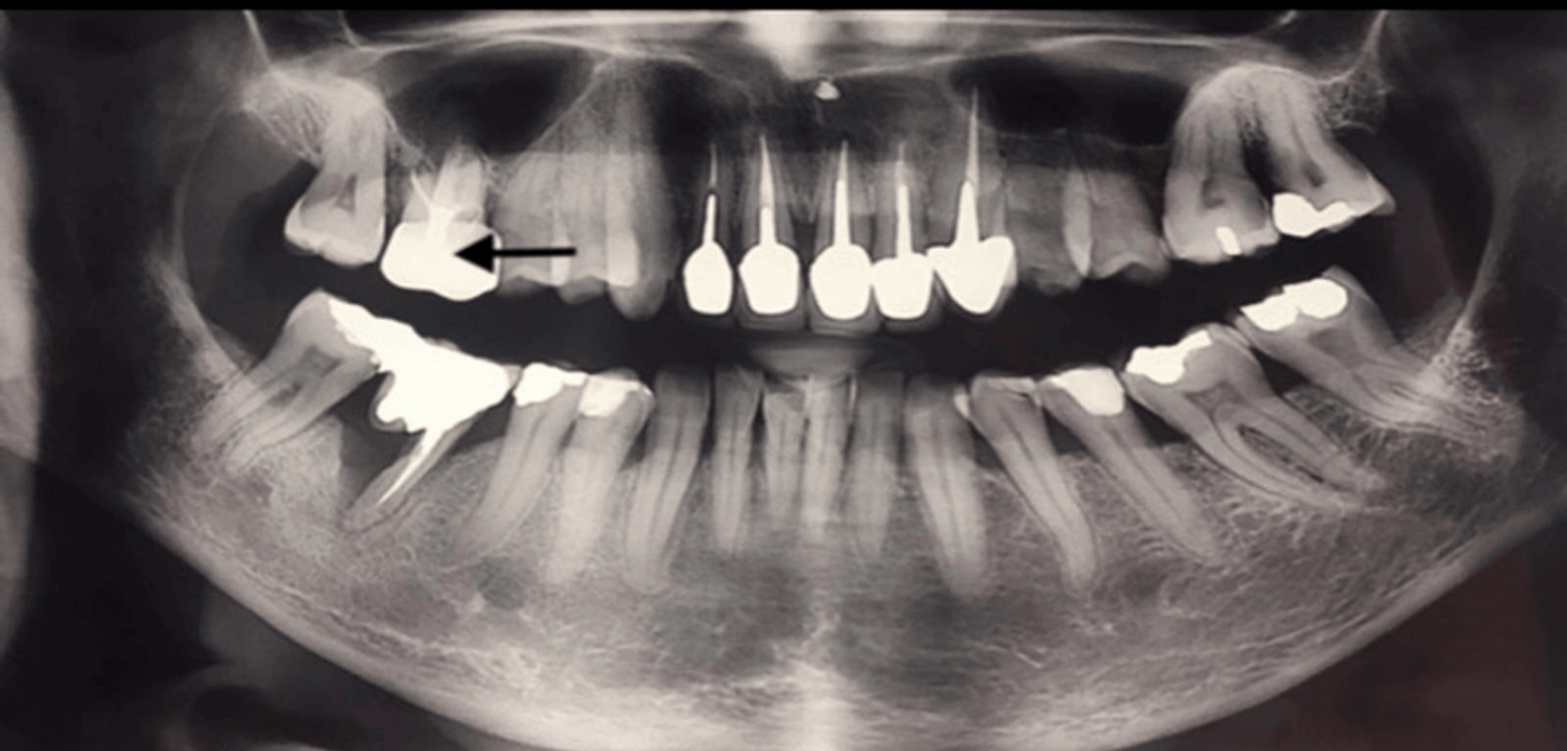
Orthopantomographic view Mobility and 50% radiographic bone loss of tooth #16 (arrow), indicating its extraction.
